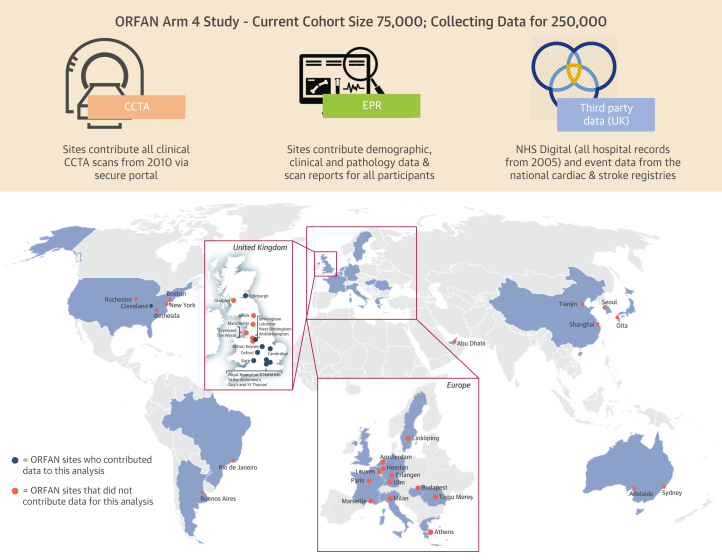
The ORFAN Arm 4 Study The ORFAN (Oxford Risk Factors and Noninvasive Imaging Study) Arm 4 study is an international multicenter retrospective cohort study of patients undergoing clinically indicated CCTA. The initial cohort size is 75,000 patients within the United Kingdom and 25,000 internationally, with ethically approved expansion underway for 250,000 patients. Within the United Kingdom, the study includes 17 National Health Service (NHS) Trusts, 4 of which contributed data to the current study. Data collected for each participant includes the CCTA scan, data from the local hospital electronic patient record (EPR), and data from authorized third parties, including NHS Digital, all hospital event data from 2005 to now; NICOR (National Institute for Cardiovascular Outcomes Research), a national cardiac event registry; and SSNAP (Sentinel Stroke National Audit Programme), a national stroke event registry. CCTA = coronary computed tomography angiography.
